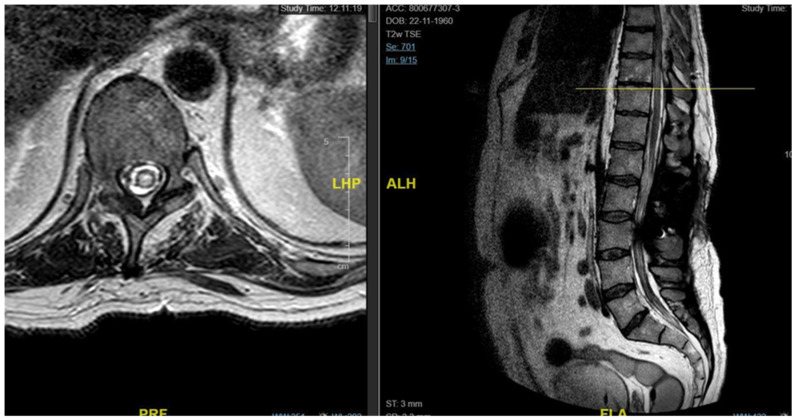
Case 3: post-operative MRI showing the absence of a recurrent cystic formation: post-operative T2 axial view (left) and post-operative T2 sagittal view (right).
